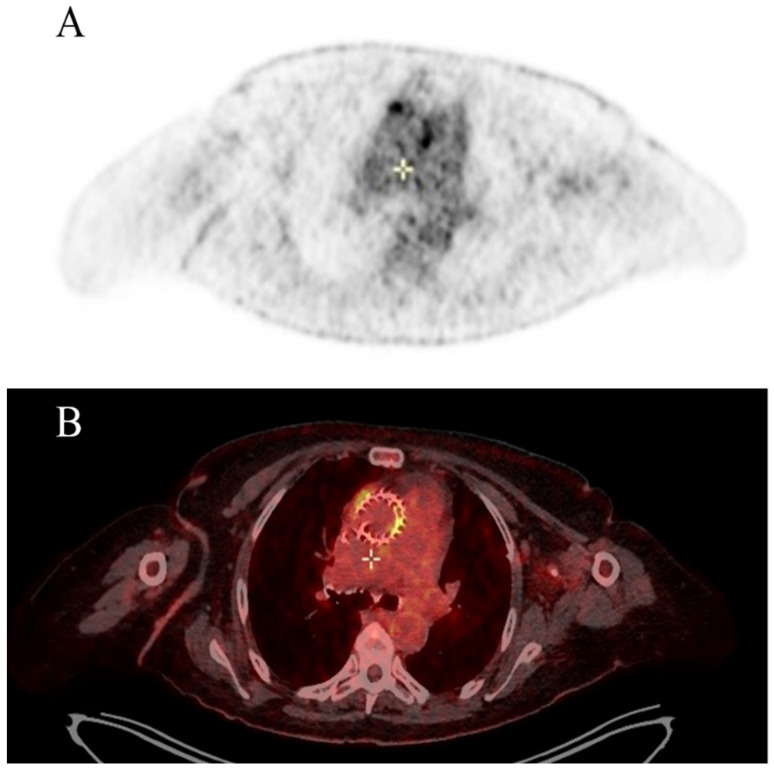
Pre-operative FDG-PET/CT imaging assessment with multifocal uptake of prosthetic transcatheter valve. (A) attenuation-corrected FDG-PET, axial slice; (B) fused attenuation-corrected FDG-PET/CT, axial slice. Abbreviation; FDG-PET/CT, fluorodeoxyglucose positron emission tomography/computed tomography.
